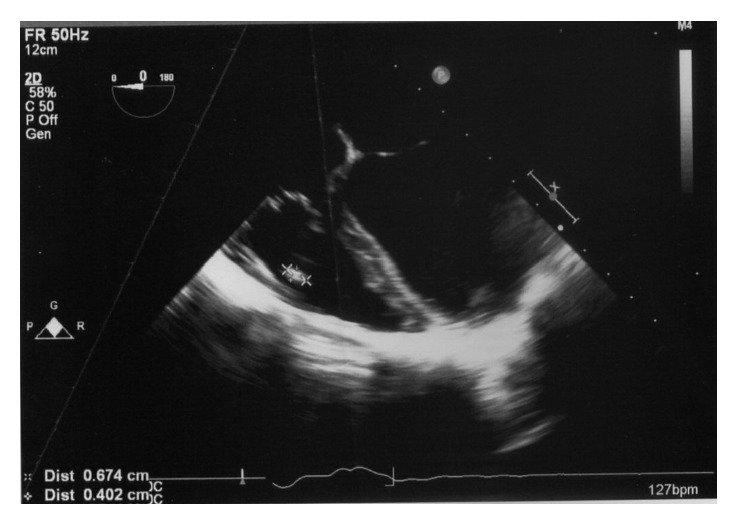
Vegetation (7 × 4 mm) seen on the tricuspid valve in transoesophageal echocardiogram (TOE).
